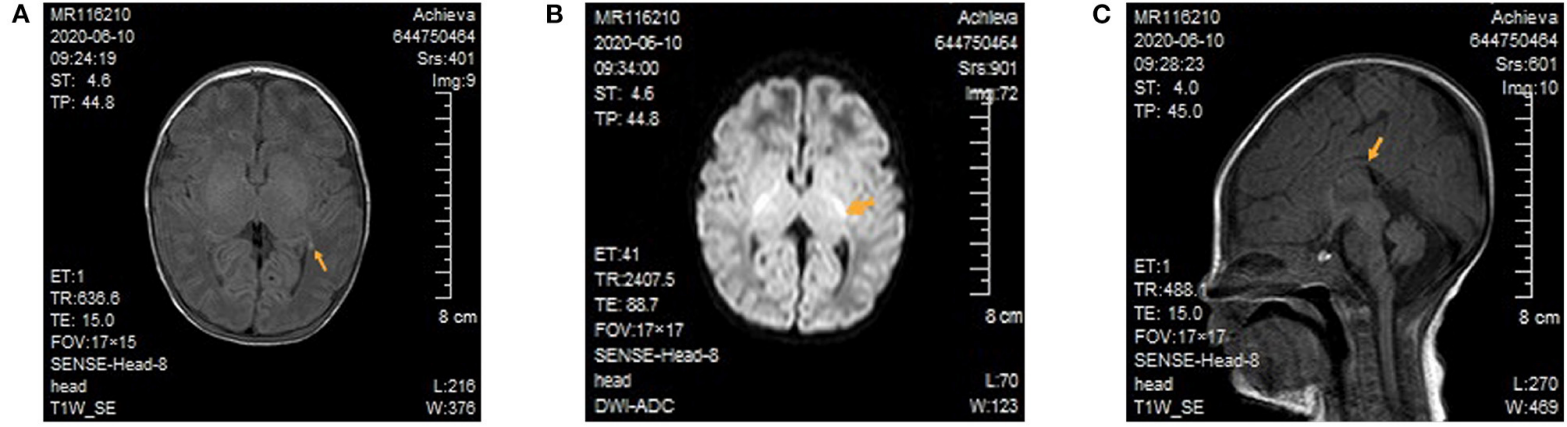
Cranial magnetic resonance imaging of the patient. (A) Focal cerebral hemorrhage of the left paraventricular brain. There was no high T1 signal in the posterior limb of bilateral internal capsule. (B) In the diffusion-weighted image, high signals can be seen for the posterior limb of the internal capsule. (C) The thin and short of corpus callosum.
